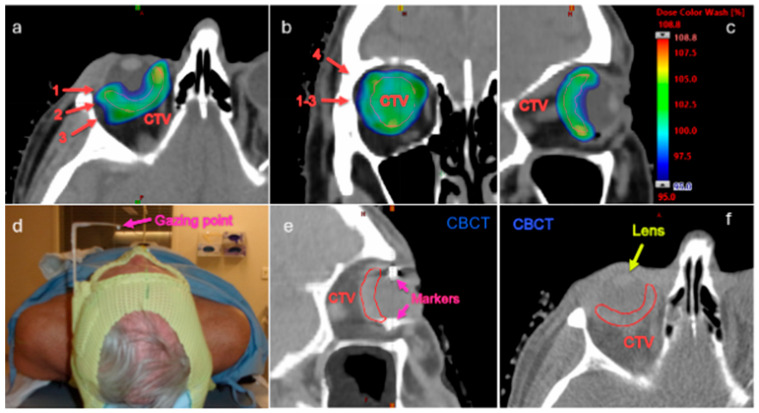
Gantry-based PBS proton treatment plan for uveal melanoma patient (a–c). The patient was planed with four fields, including one non-coplanar beam (red arrows). The patient was treated in a supine position with a gaze fixation device (d). Imaging guidance includes CBCT alignment to the surrounding bones, tantalum markers, and visible anatomies (e,f). CTV = clinical target volume. CBCT = cone beam computed tomography.
